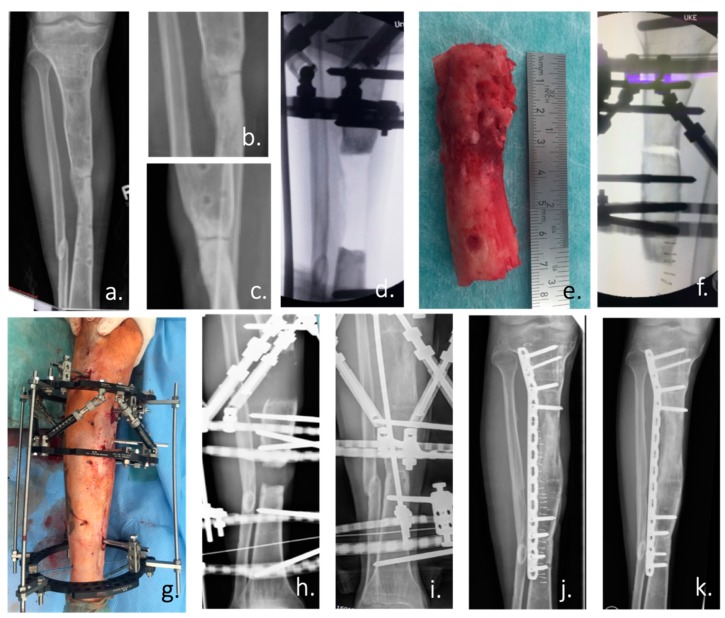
(a) Ap radiograph of the right lower leg of a 24-year-old man with an atrophic non-union of the tibia after an open fracture in Albania 5 years ago. (b,c) Enlargement of the non-union in an ap and lateral radiograph. According to the patient’s history: condition after 4 surgical trials in 3 countries. (d) Intraoperative ap radiograph after positioning of the Taylor Spatial Frame (TSF) external fixator and radical resection of the atrophic non-union. (e) Resected tibia non-union with atrophic bone over a length of 7 cm. (f) Intraoperative positioning of the tibia and the TSF for the planned bone segment transport by callus distraction. (g) Postoperative picture of the attached TSF. (h) Radiograph of the tibia during the distraction process (0.5 mm/day over a period of 140 days) with constant substitution of calcium and vitamin D. (i) X-ray of the tibia after the end of the distraction with already clearly visible bone formation. (j) Postoperative radiograph after removal of the ring fixator and angular stable plate osteosynthesis. (k) Radiological control at the end of treatment with healed bone defect 3 months after TSF removal.
